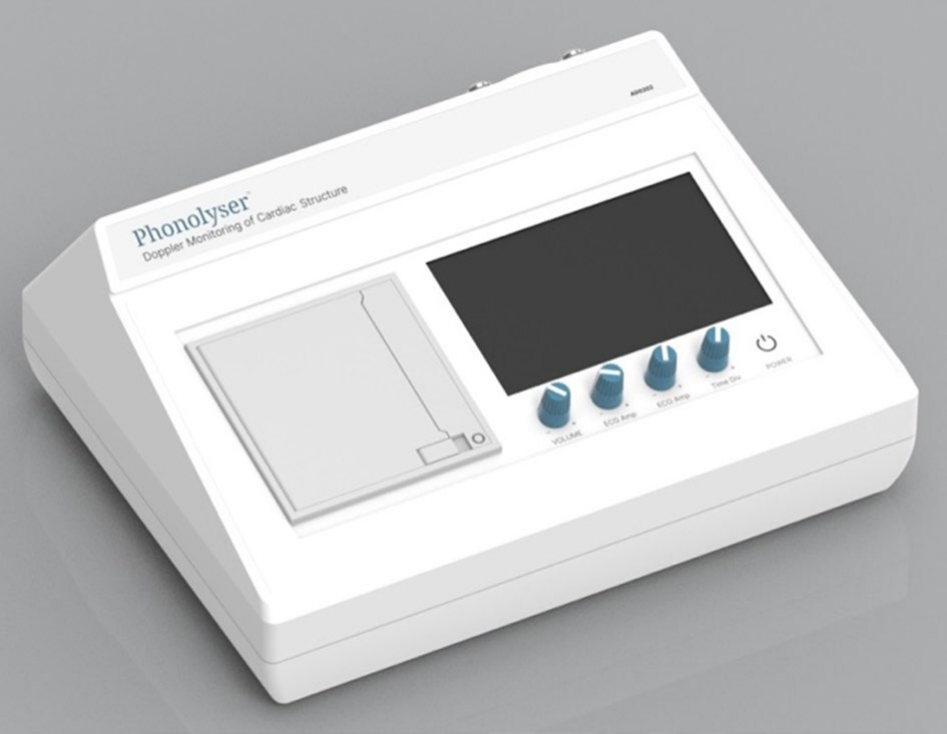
Doppler Phonolyser device
